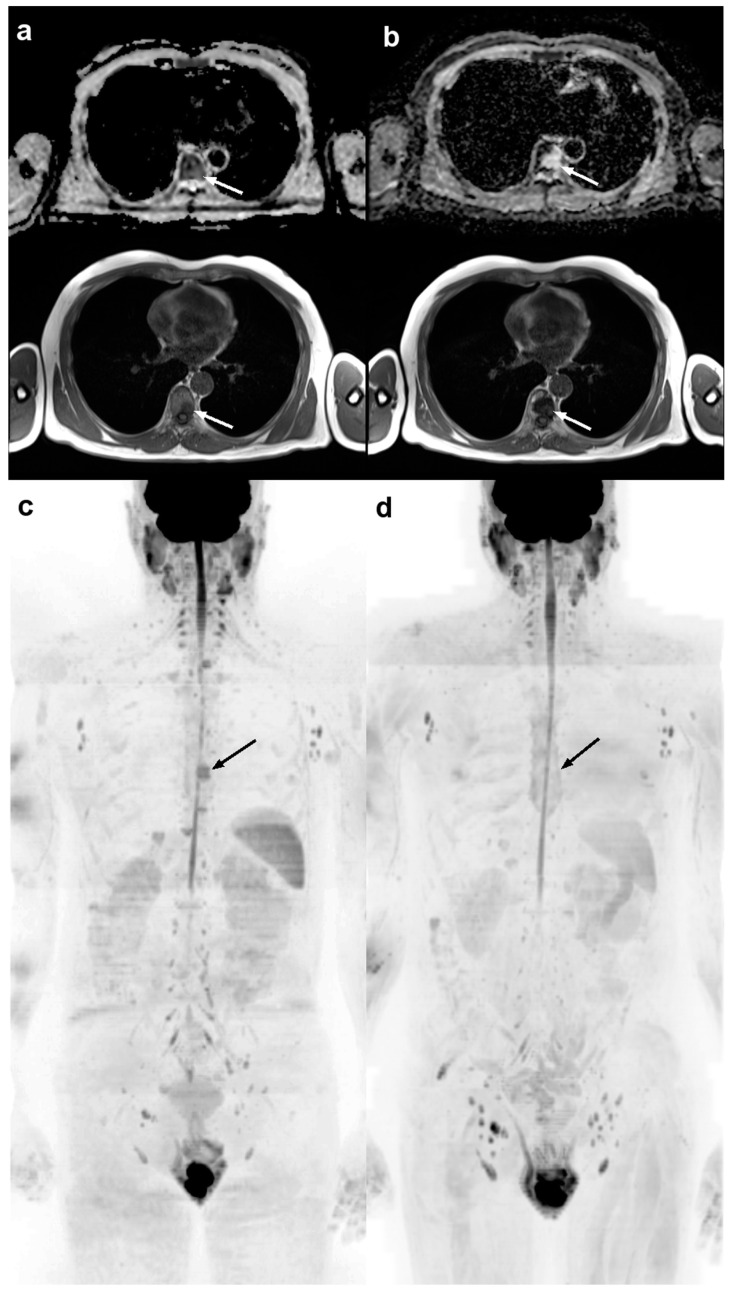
Whole-body magnetic resonance images of a 67-year-old man with metastatic hormone-sensitive prostate cancer showing response to treatment, with primary/dominant Response Assessment Category (RAC) of 1. (a) Axial apparent diffusion coefficient (ADC) map (upper) and T1-weighted (lower) images at the start of luteinizing hormone releasing hormone agonist therapy show the presence of a dorsal spine lesion (arrows) with ADC value of 784 μm^2^/s. (b) Axial ADC map (upper) and T1-weighted (lower) images at the follow-up show an increase in the spine lesion size accompanied by an increase in the ADC value (1608 μm^2^/s), suggestive for edema. Thus, this picture was classified as indicative of a highly likely response. Three-dimensional b900 maximum intensity projection images (c) at start of therapy and (d) at follow-up confirm the disappearance of the spine lesion. Reprinted adapting the caption from Pricolo et al. [29] under the terms and conditions of the Creative Commons Attribution 4.0 International License.
